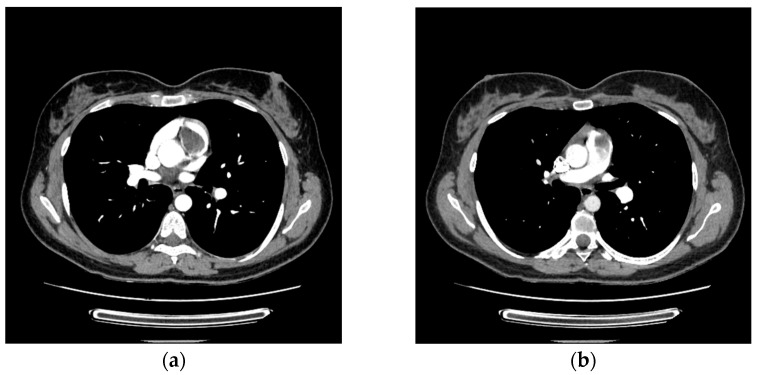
Pulmonary CT angiography displaying the tumoral mass: (a) in the right ventricular outflow tract; (b) in the main pulmonary artery.
